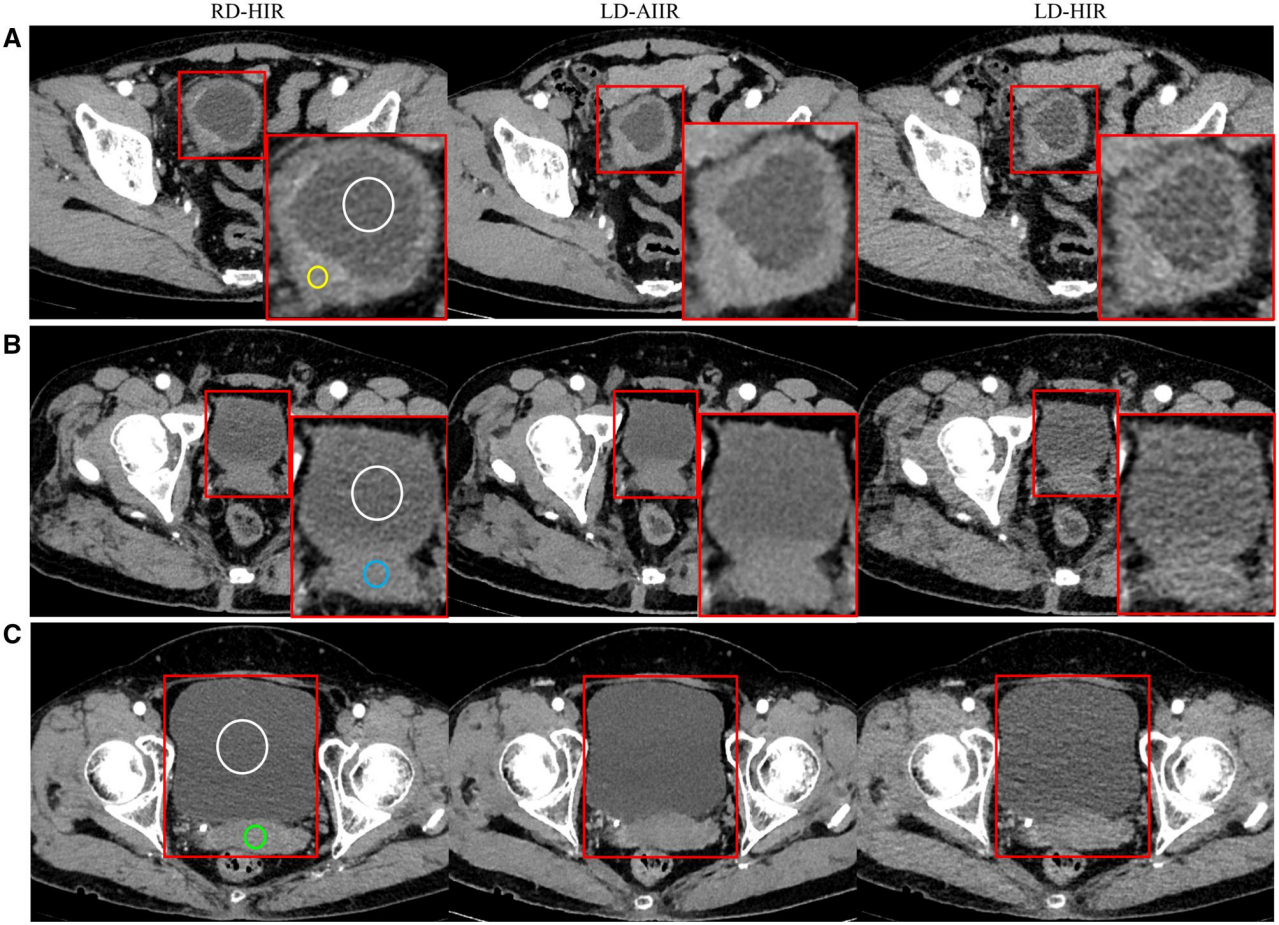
Corticomedullary phase CT images of (A) a 69-year-old man, (B) a 55-year-old man and (C) a 58-year-old woman at routine-dose with hybrid iterative reconstruction (RD-HIR), low-dose with artificial intelligence iterative reconstruction (LD-AIIR)and with hybrid iterative reconstruction (LD-HIR), where the contrast-to-noise ratio is shown for the (A) bladder wall (yellow) and lumen (white), (B) bladder lumen (white) and prostate (blue), and (C) bladder lumen (white) and uterus (green).
